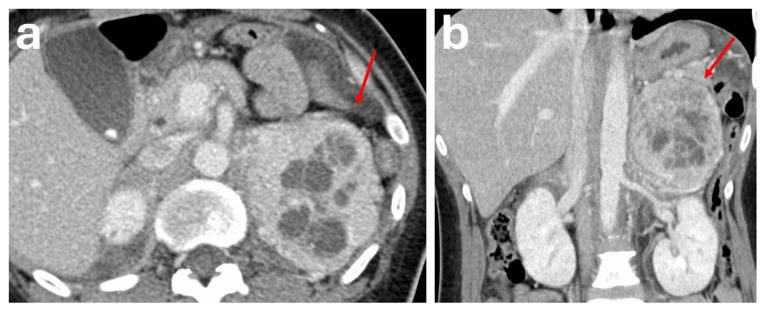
Patient with a history of hypertension and unexplained weight loss over a 12-month period had a CT TAP performed for malignancy screening. The CT demonstrated a large 9.0 × 8.7 × 7.0 cm heterogeneous mass in the left suprarenal region (red arrow): (a) axial plane, (b) coronal plane. The solid areas of the mass showed avid enhancement on the portal venous phase (HU 140). Internal areas of non-enhancement represent necrosis. The mass displaced the adjacent pancreatic tail and splenic flexure with no evidence of invasion. Biochemical testing showed raised urine and plasma metanephrines. This was proven to be a phaeochromocytoma post-resection. Generic testing showed a pathogenic variant in the MAX gene (MYC-associated factor X), associated with hereditary paraganglioma–phaeochromocytoma (PGL-PCC) syndrome.
